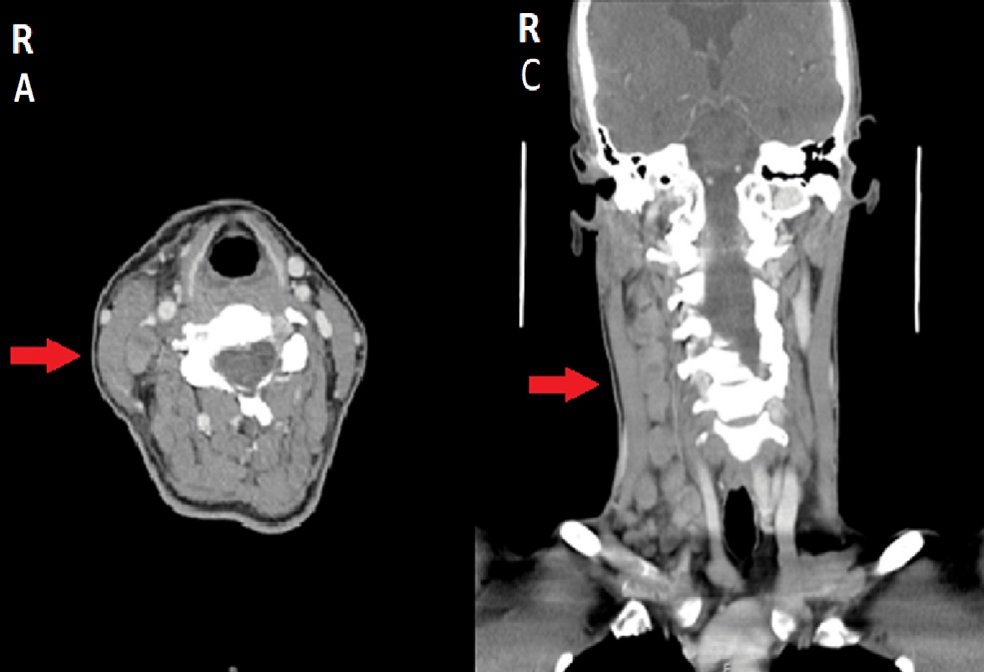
CT of the neck with adenopathies in various ganglionar levels in the right neck R – Right side; A - Axial section CT scan;  C - Coronal section CT scan;  Red arrow points to adenopathy
